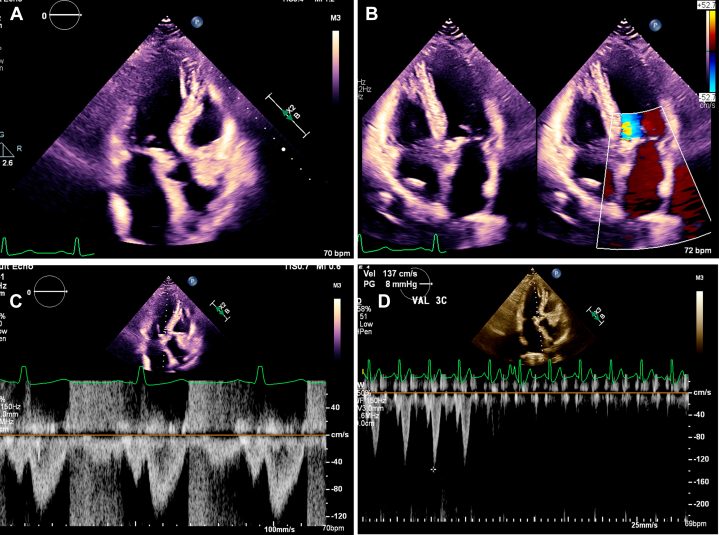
TTE After 12 Months of Mavacamten Therapy Demonstrating Stable and Decreased LVOT Gradients and a Reduction in MR (A) Apical 3-chamber view with the degree of systolic anterior motion reduced compared to prior. (B) Apical 4-chamber view with no significant MR. (C) LVOT resting pulsed-wave Doppler without aliasing and a peak velocity of 1.2 m/s (D) LVOT pulsed-wave Doppler during Valsalva maneuver with a gradient of 8 mm Hg and maneuver release showing a decrease in the velocity. LVOT = left ventricular outflow tract; MR = mitral regurgitation; TTE = transthoracic echocardiography.
